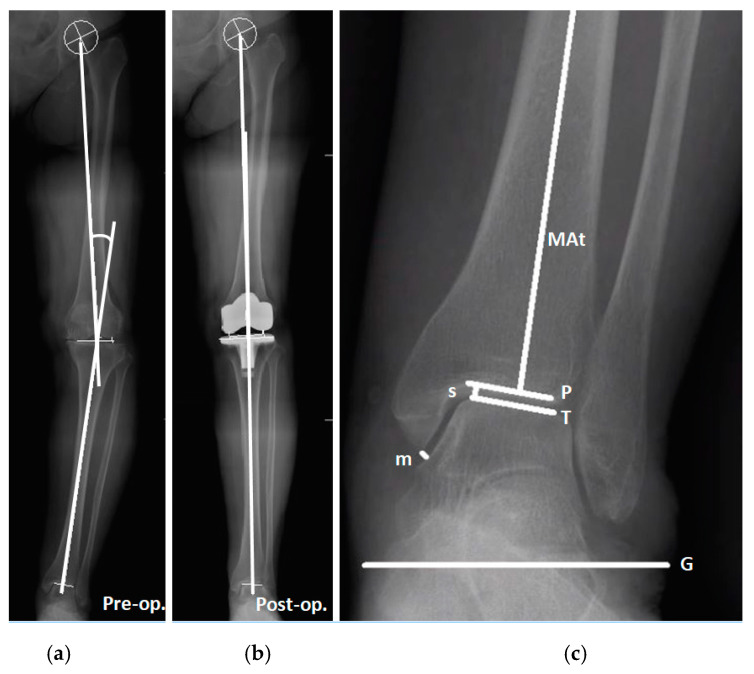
Preoperative and postoperative measurement of radiographic parameters for the knee and ankle in one patient. (a) Preoperative HKA measurement. Positive HKAs denote varus knee alignment, whereas negative HKAs denote valgus knee alignment. (b) Postoperative HKA measurement. (c) Ankle parameters: MAt line, mechanical axis of the tibia; P line, tibial plafond; T line, talar dome; G line, ground line. The LDTA is defined as the lateral angle of the MAt line and the P line. The PGA is defined as the angle between the P line and the G line. The TGA is defined as the angle between the T line and the G line. The PTA is defined as the angle between the P line and the T line. Positive PGA, TGA, and PTA values mean that the angle opens outward, whereas negative values mean that the angle opens inward. The MS and SS are indicated by m and s, respectively.
